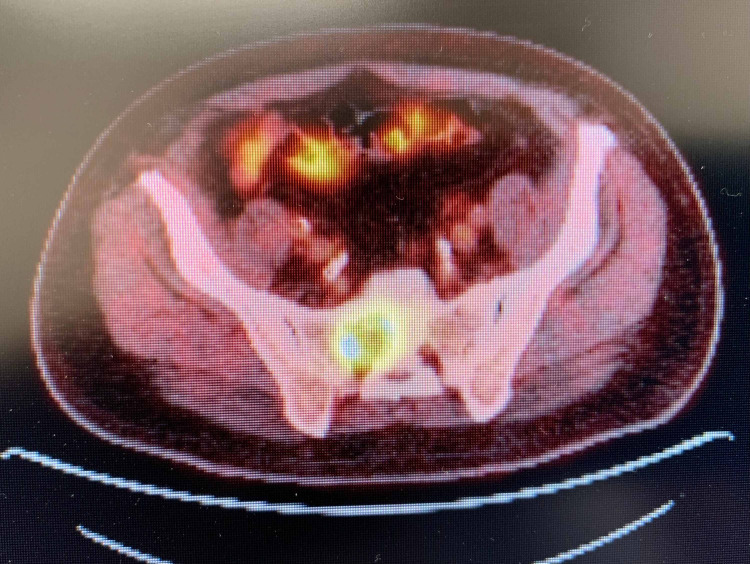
PET scan A large lytic lesion is identified at the right sacrum, with a maximum SUV of 11.4 SUV: standardized uptake value; PET: positron emission tomography
